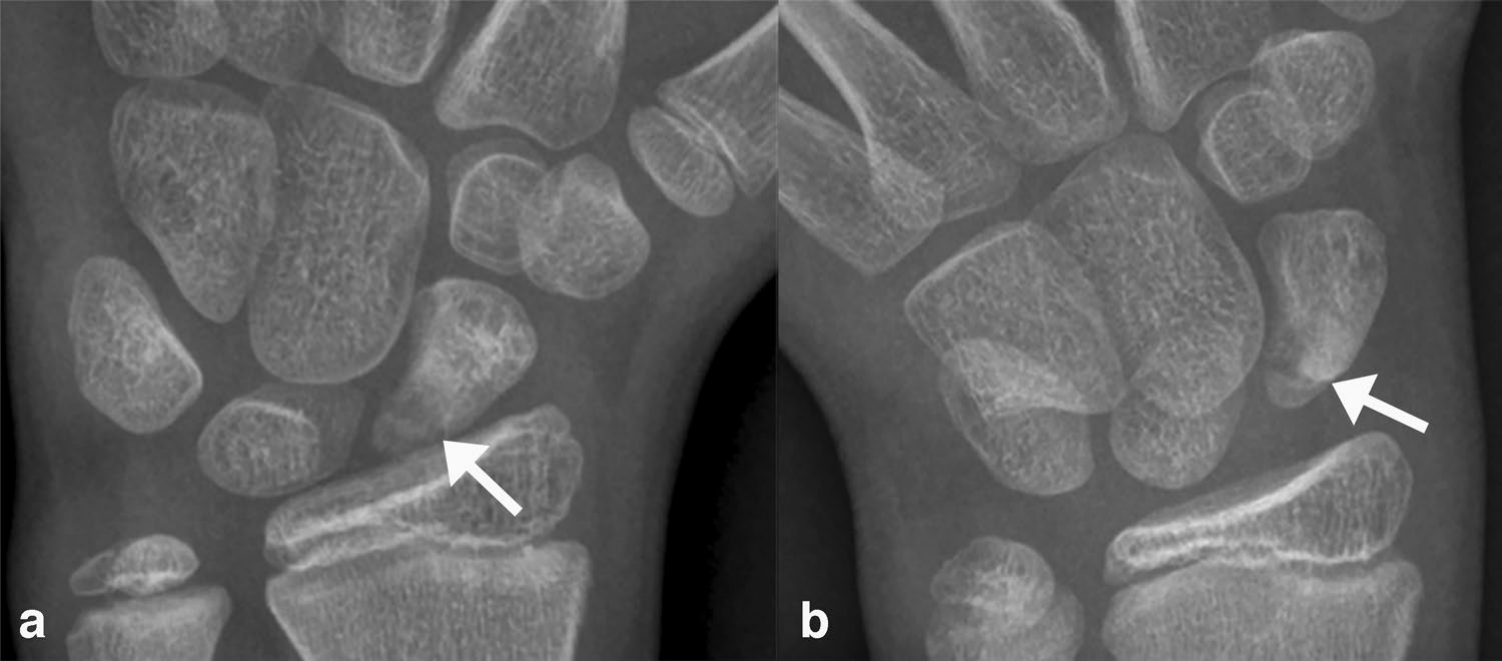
Intraarticular scaphoid fracture in a skeletally immature 11-year-old boy. Posteroanterior (PA, a) and PA scaphoid (b) radiographic views show a fracture (arrows) of the proximal pole that extends into the radioscaphoid joint. The patient underwent open reduction and internal fixation (ORIF) with a distal radius autograft
